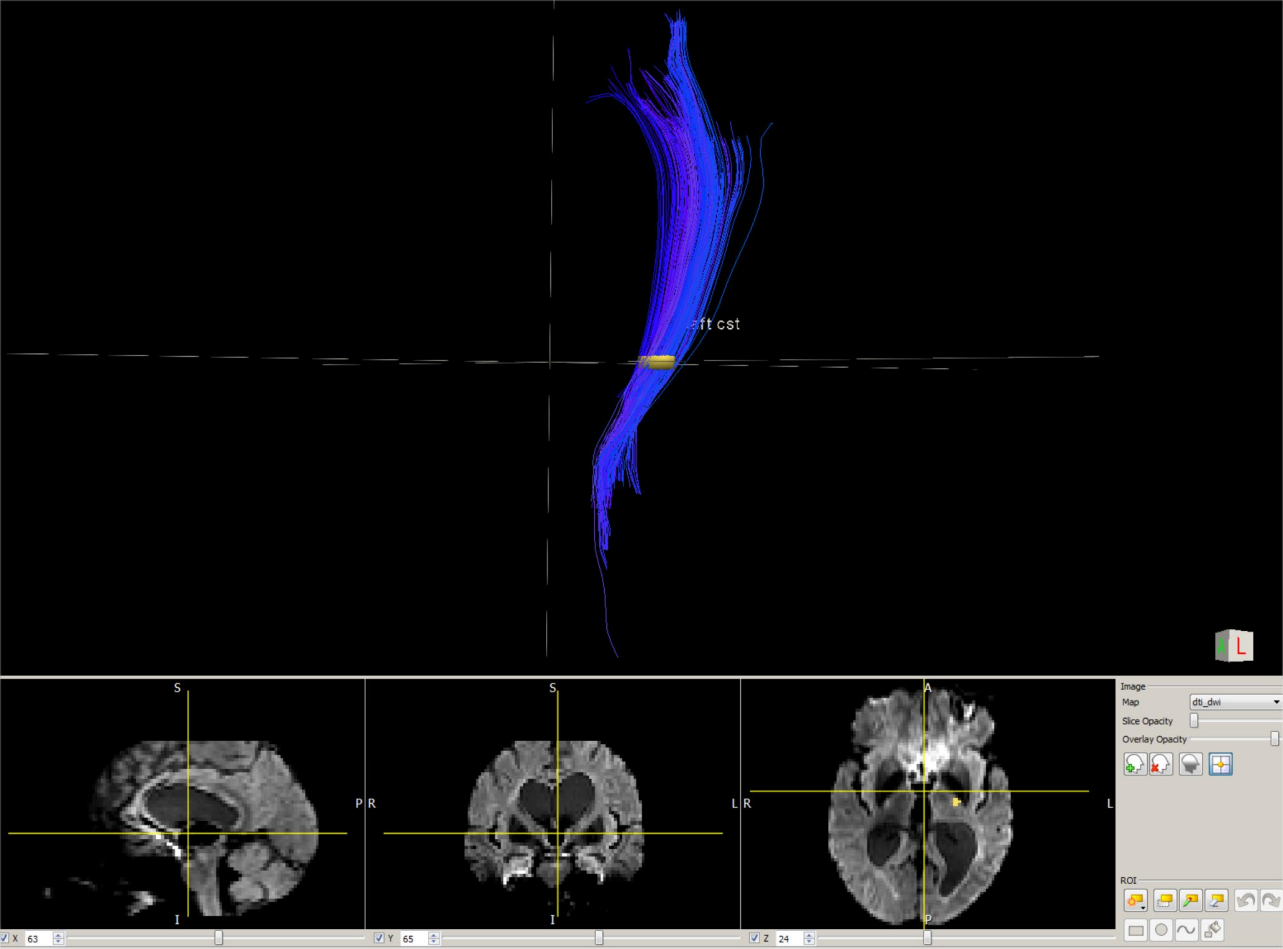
Example of magnetic resonance imaging tractography of left corticospinal tract (CST) (blue, upper image) performed from posterior internal capsule ROI (yellow cross, the lower images) in a 43-year-old patient with secondary progressive multiple sclerosis. The measures were as follows: fractional anisotropy (FA) 0.6, apparent diffusion coefficient (ADC) 0.1, Axial diffusivity (AD) 0.2, radial diffusivity (RD) 0.06, and volume 7 mL
